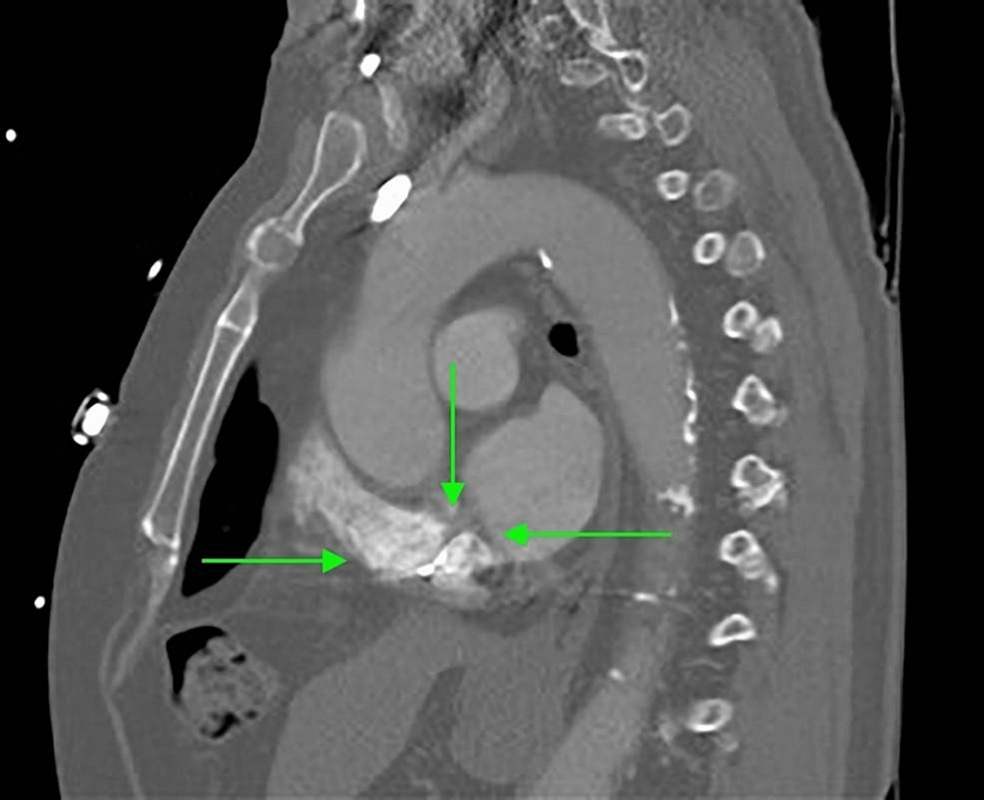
CTA of the chest, sagittal section, revealing small intra-pericardial hernia CTA: computed tomography angiogram.
